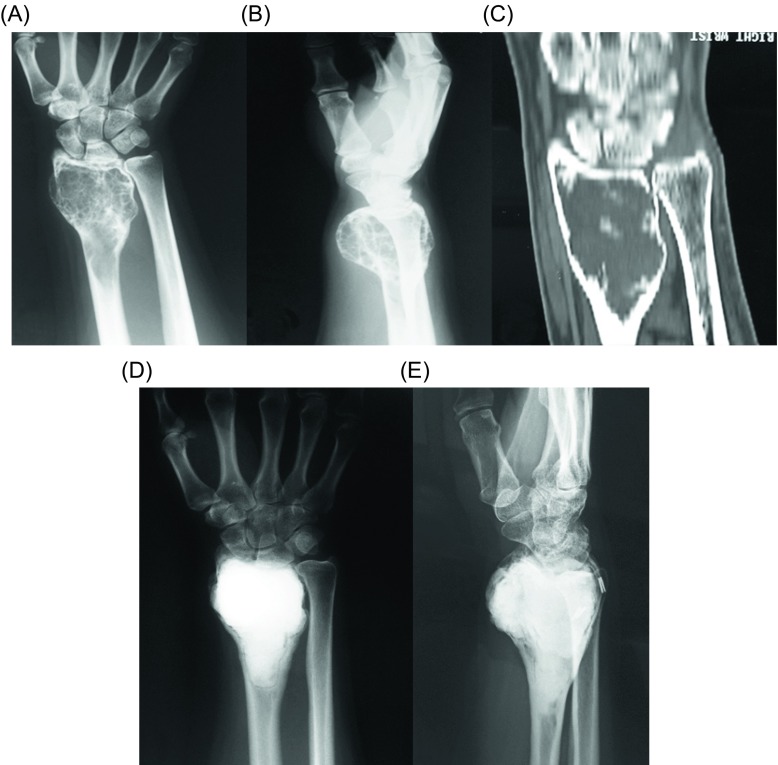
(A) Anteroposterior and (B) lateral radiographs, and (C) coronal CT of the right wrist of a 43-year-old man with a GCT of the distal radius. Curettage, cauterization, and PMMA cementation were done. (D) Anteroposterior and (E) lateral radiographs of the right wrist show no evidence of local tumor recurrence at eight-year follow-up.
